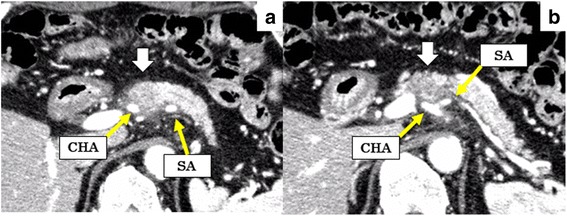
Abdominal CT scan after two courses of GS. The white arrows indicate the pancreatic tumor. a The size of the main tumor was reduced from 26 mm to 21 mm. The abnormal shadow of the soft tissue around CHA did not increase. b The abnormal shadow around the bifurcation of Ce did not change. CHA common hepatic artery, Ce celiac artery, SA splenic artery
